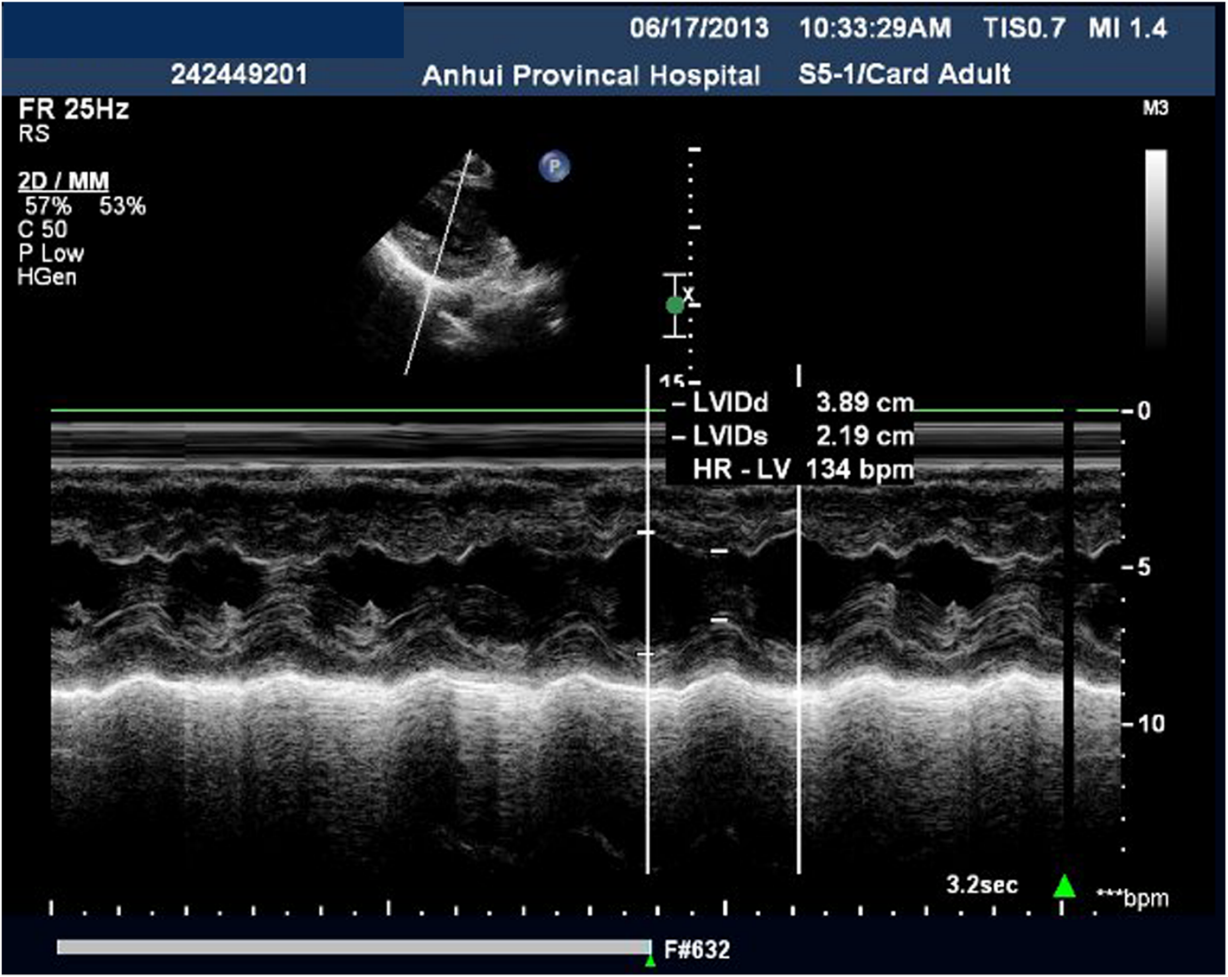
Photo of an echocardiographic Doppler flow velocity measurement from the level of the aortic annulus from the parasternal long-axis window.
